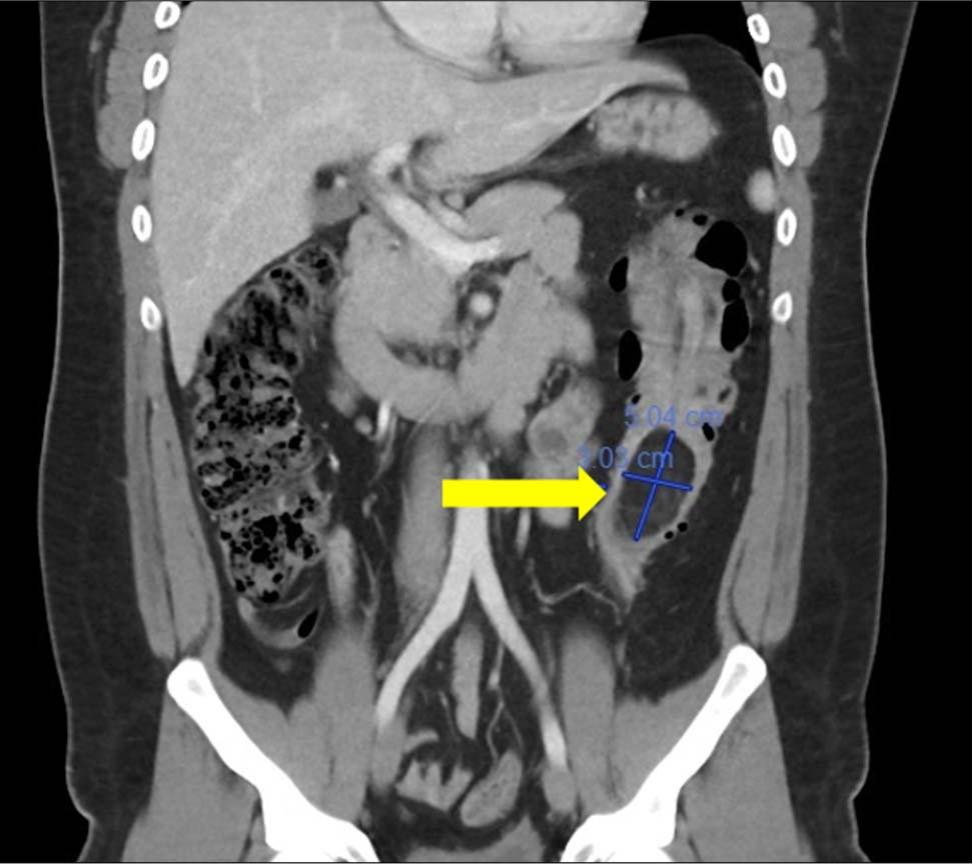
Contrast enhanced CT, coronal view showing intussuscepting colonic lipoma in the descending colon (yellow arrow). CT, computed tomography.
